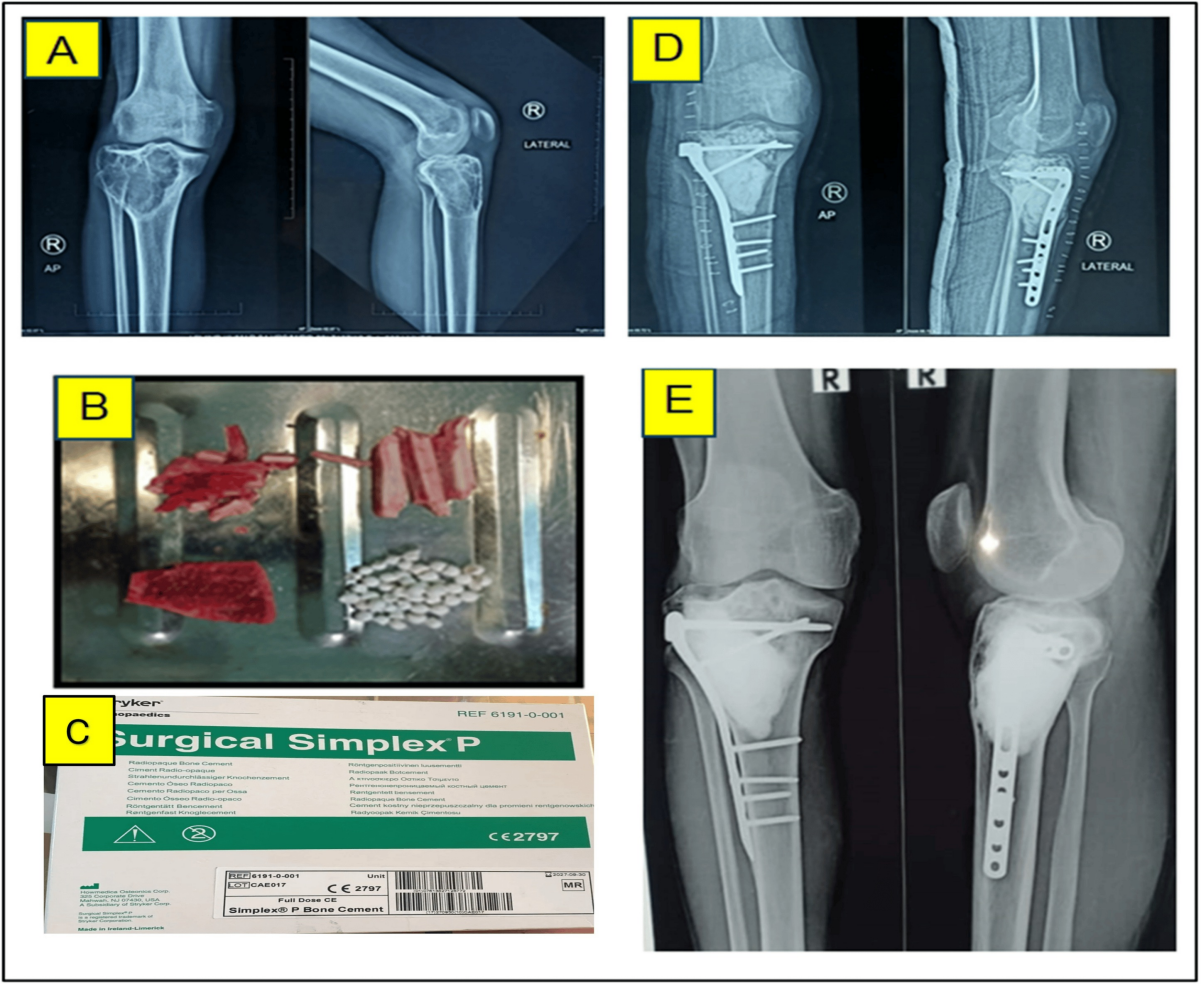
Extended Curettage (EC) in a 32-year-old male. (A) Preoperative X-ray of right proximal tibia showing giant cell tumor (GCT); (B) Intraoperative image depicting iliac crest graft, fibular bone graft, and artificial bone substitute; (C) Bone cement; (D) Postoperative X-ray of the right knee; (E) Four-year follow-up X-ray demonstrating new bone formation at the subarticular surface of the right proximal tibia
